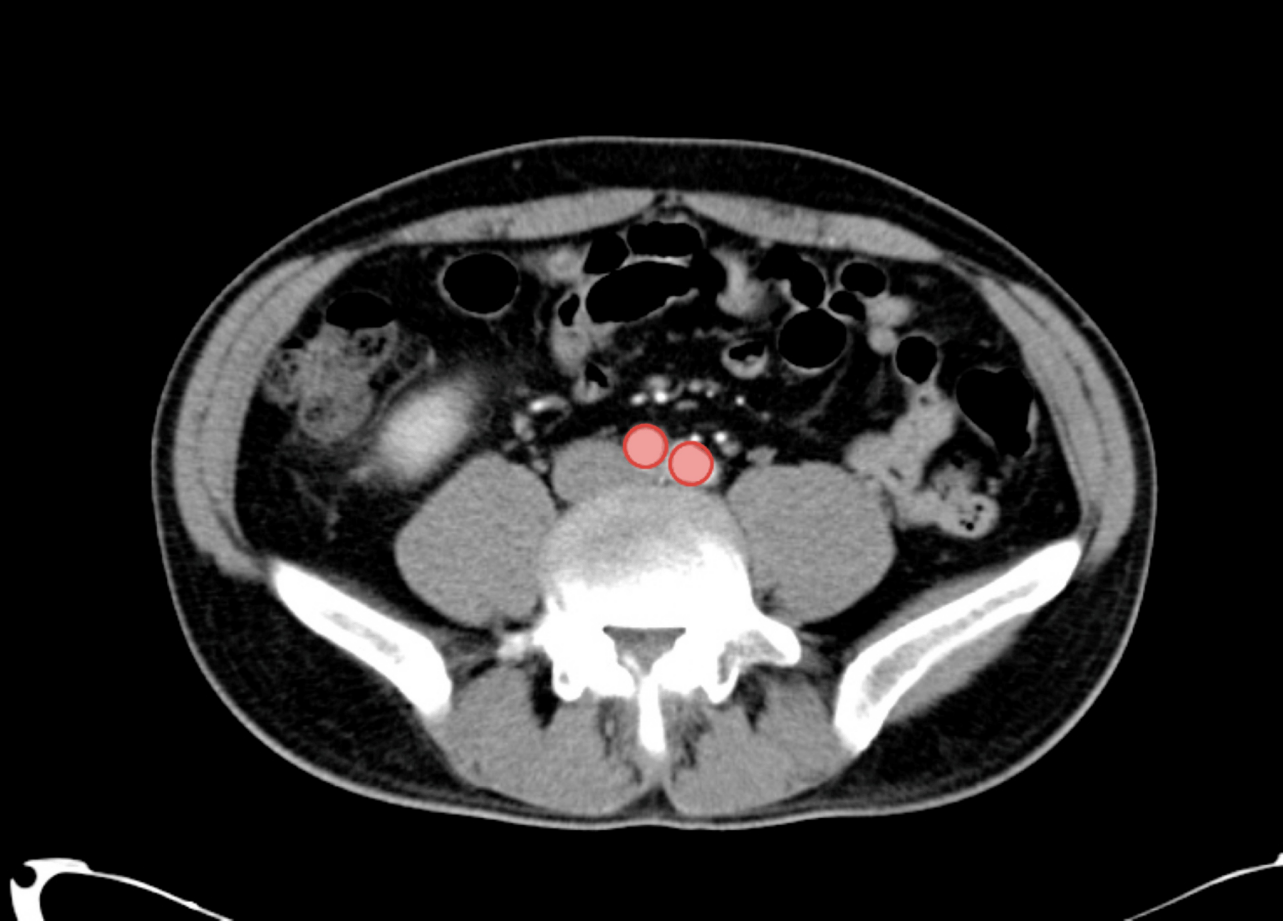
CT scan in the axial view showing the bifurcation of the aorta = level of L4 (red circles).
